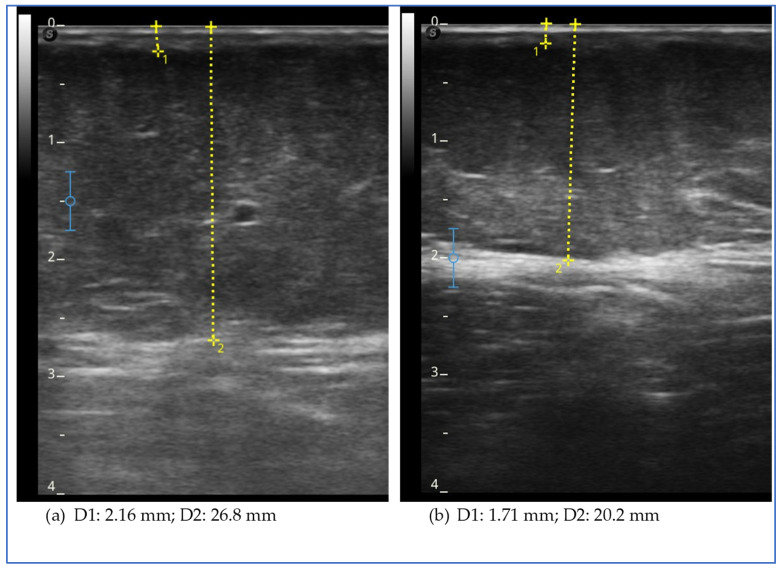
Ultrasound measurements for case report 5.
